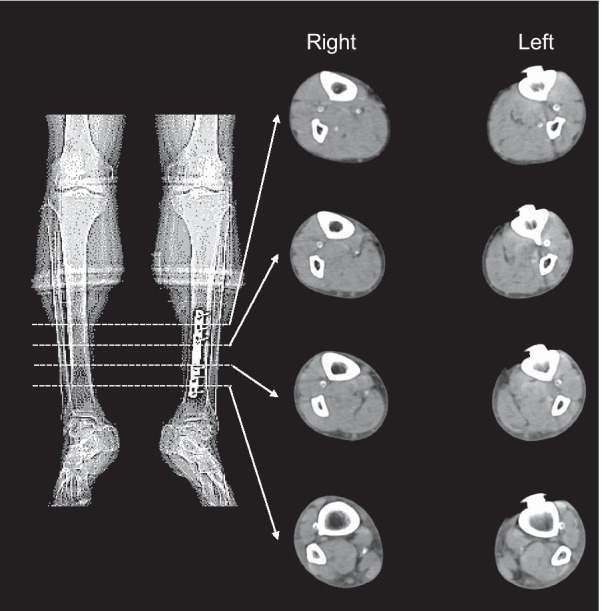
Computed tomography of the lower legs. Axial images of plain computed tomography showed severe calcification of infrapopliteal arteries in the bilateral lower legs
